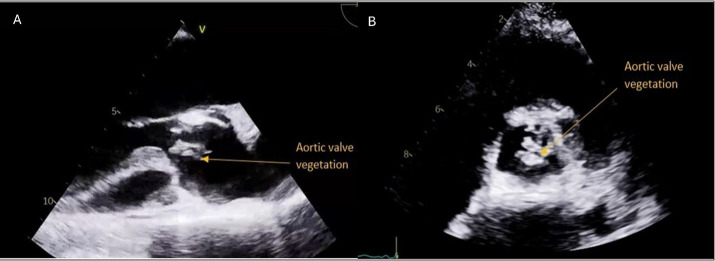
Transthoracic echocardiogram, A-parasternal long axis view, B-parasternal short axis view showing echogenic mass on the aortic valve (arrows), representative of aortic valve vegetation.
